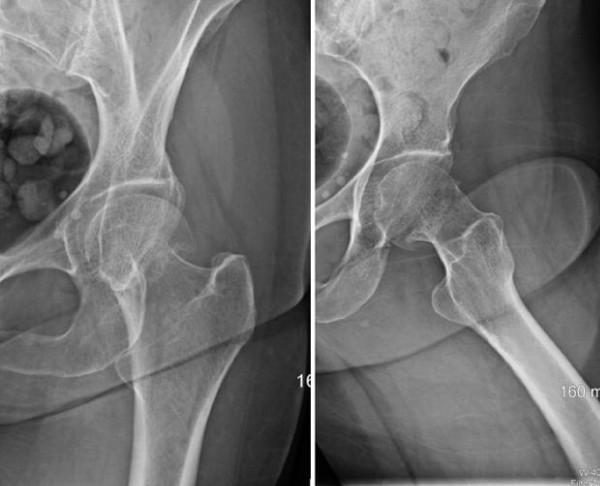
Case 1: preoperative radiographs of the left hip (anteroposterior and lateral views) demonstrating the impacted femoral neck fracture.
